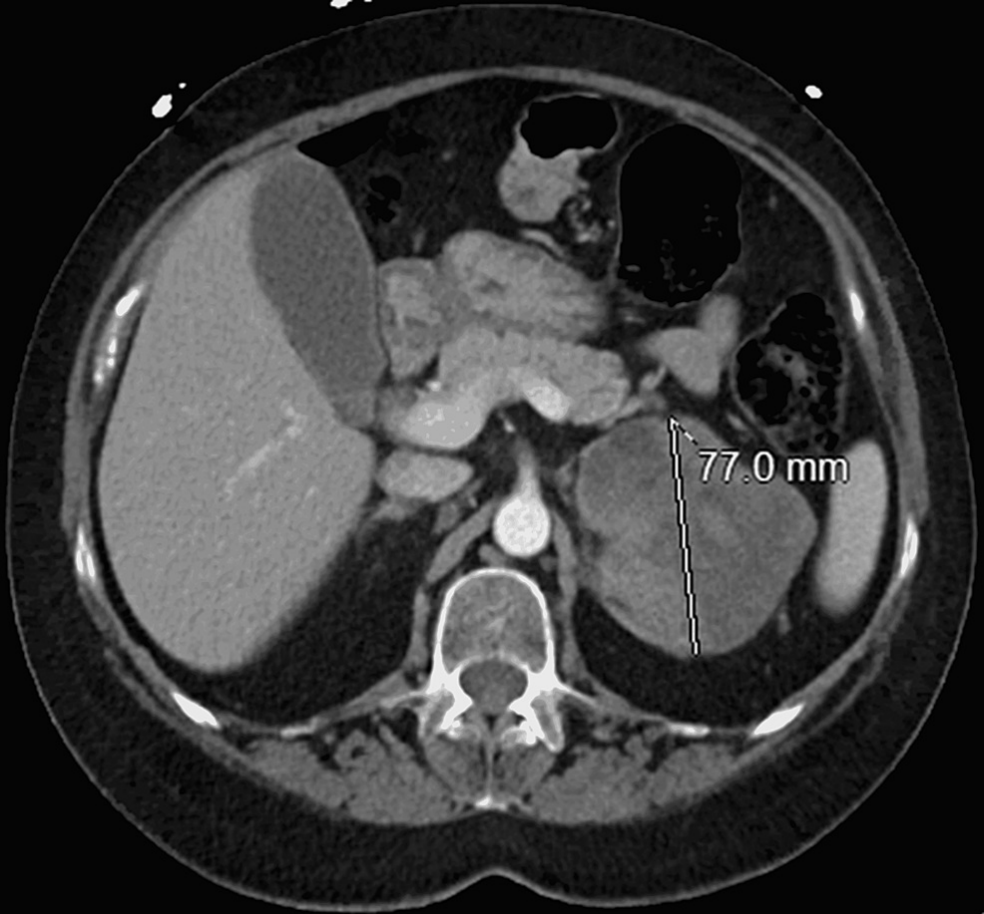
Computed tomography image of the abdomen showing adrenal mass on axial plane Image showing heterogenous 7.7 cm lesion (white line measure) appearing to be arising from left adrenal glands in contact with the upper pole of left kidney with areas of hypodensity suggesting necrosis.
